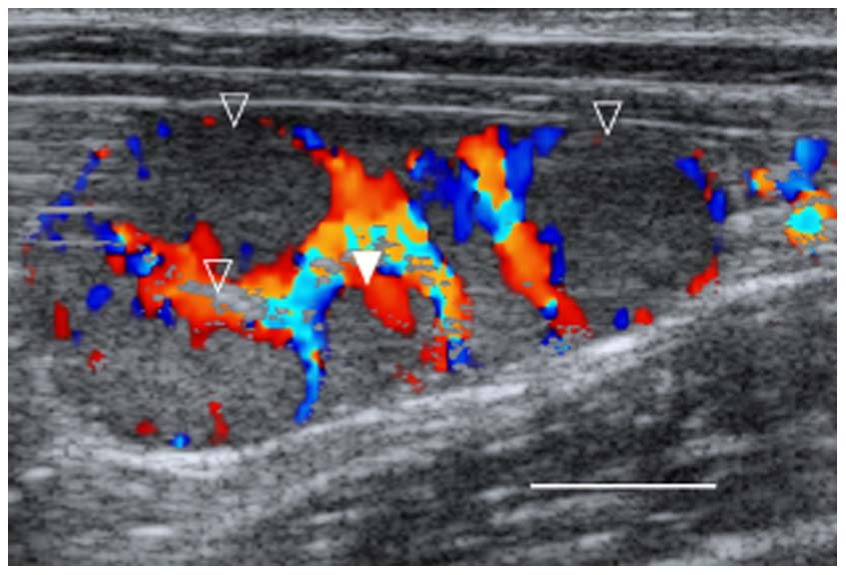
Persistent *corpora lutea* (CLs) of lynx in ultrasonography. Prolonged di-estrus in lynx outside breeding season in December. Empty triangle points at CLs from this years' ovarian cycle. Full triangle points out one old CL, at least two years old.
